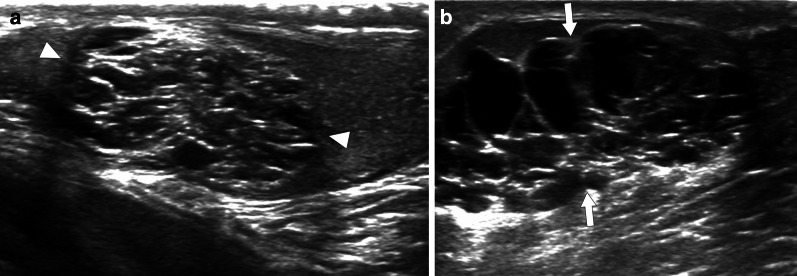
Cystic dysplasia of the rete testis in two different patients with known ipsilateral multicystic dysplastic kidney. a A 3-year-old boy with palpable right testicular mass. Gray scale testis US showed well-defined multicystic lesions within the right mediastinum testis. The lesion contains serpiginous tubular anechoic areas with intervening echogenic septations (arrowheads). No internal vascularity was detected on color flow Doppler US exam (not shown). Cystic dysplasia of rete testis was considered due to patient demographics and history. The lesion was stable on his last follow-up US exam 2 years after initial presentation. b A 7-year-old boy presenting with left testicular swelling. Gray scale US image shows a large multicystic lesion almost completely replacing the left testis parenchyma (arrows). Again the patient demographics, history and imaging findings were consistent with cystic dysplasia of the rete testis. Findings were stable on serial follow-up US exams
